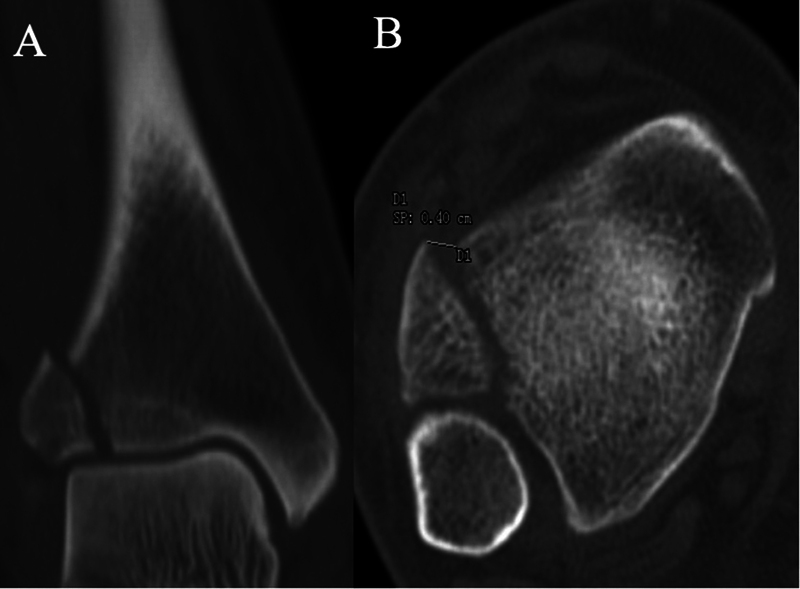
Preoperative CT scan view: (
